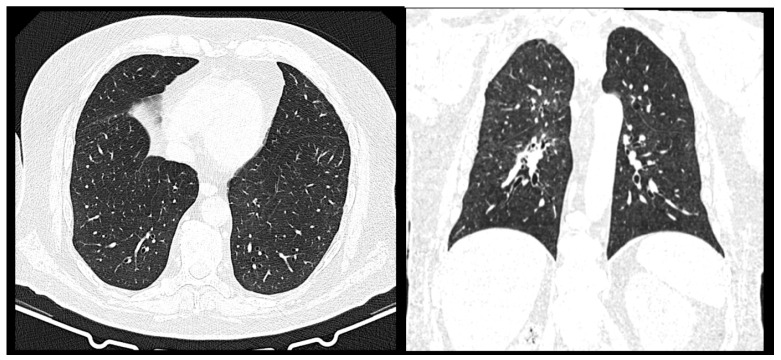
CT scan of the thorax—December 2024. No newly occurring or evolutionary pleuro-pulmonary nodular lesions.
